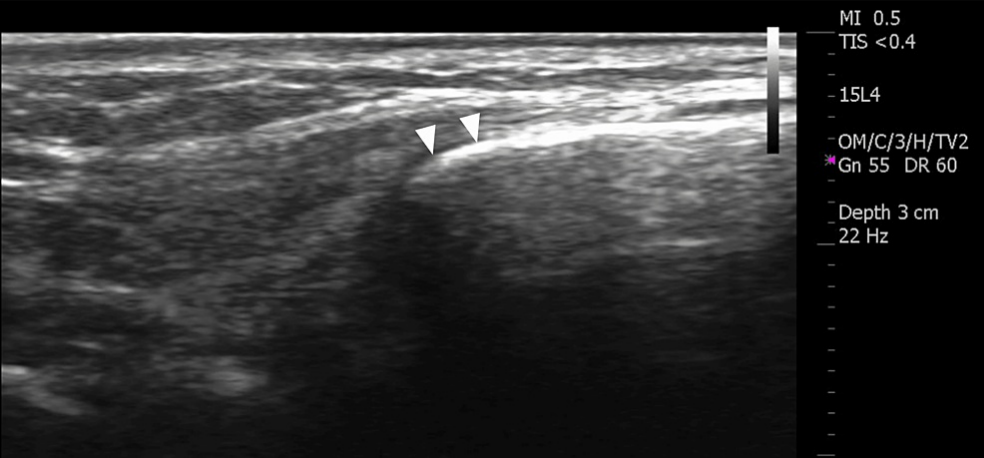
Longitudinal gray scale ultrasound view of the base of the asymptomatic, contralateral third metacarpal (arrowheads) for comparison. The left side of the image is proximal.
